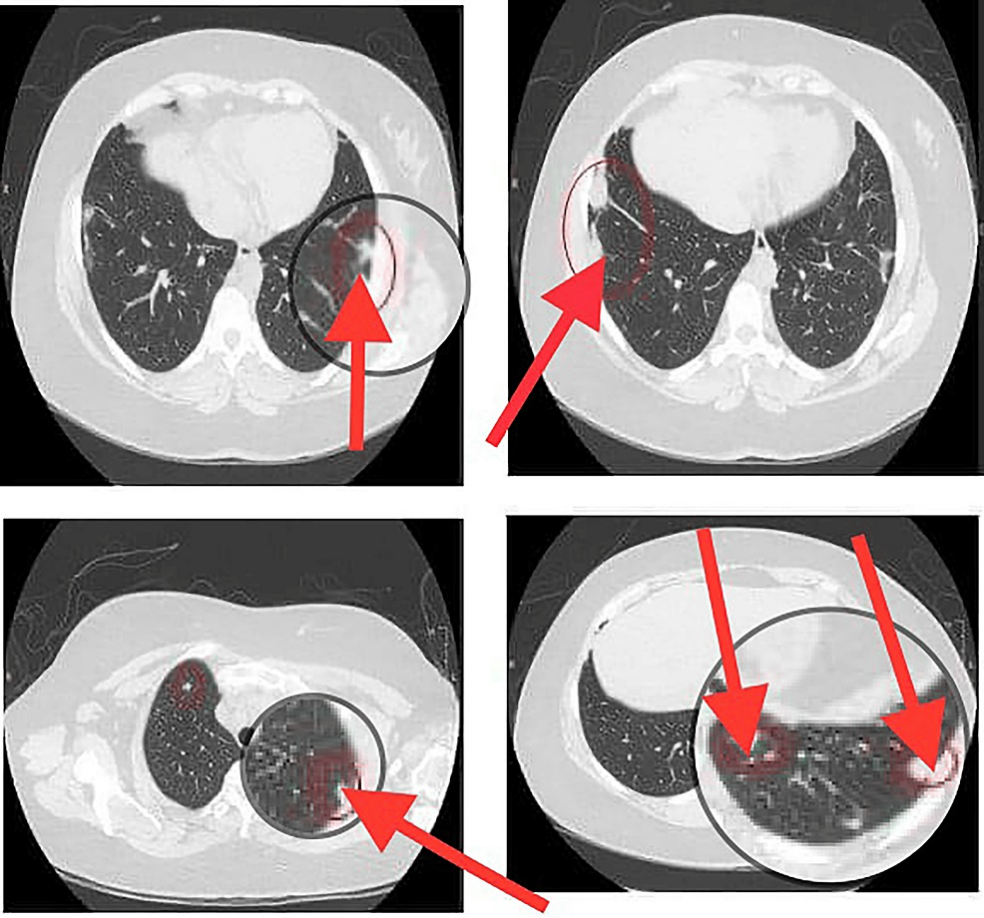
Computed tomography (CT) of the thorax revealing multiple variably sized, ill-defined pulmonary lesions/nodules at the periphery of both lower lobes, along with small nodules observed at the periphery of both upper lobes (July 23, 2023).
